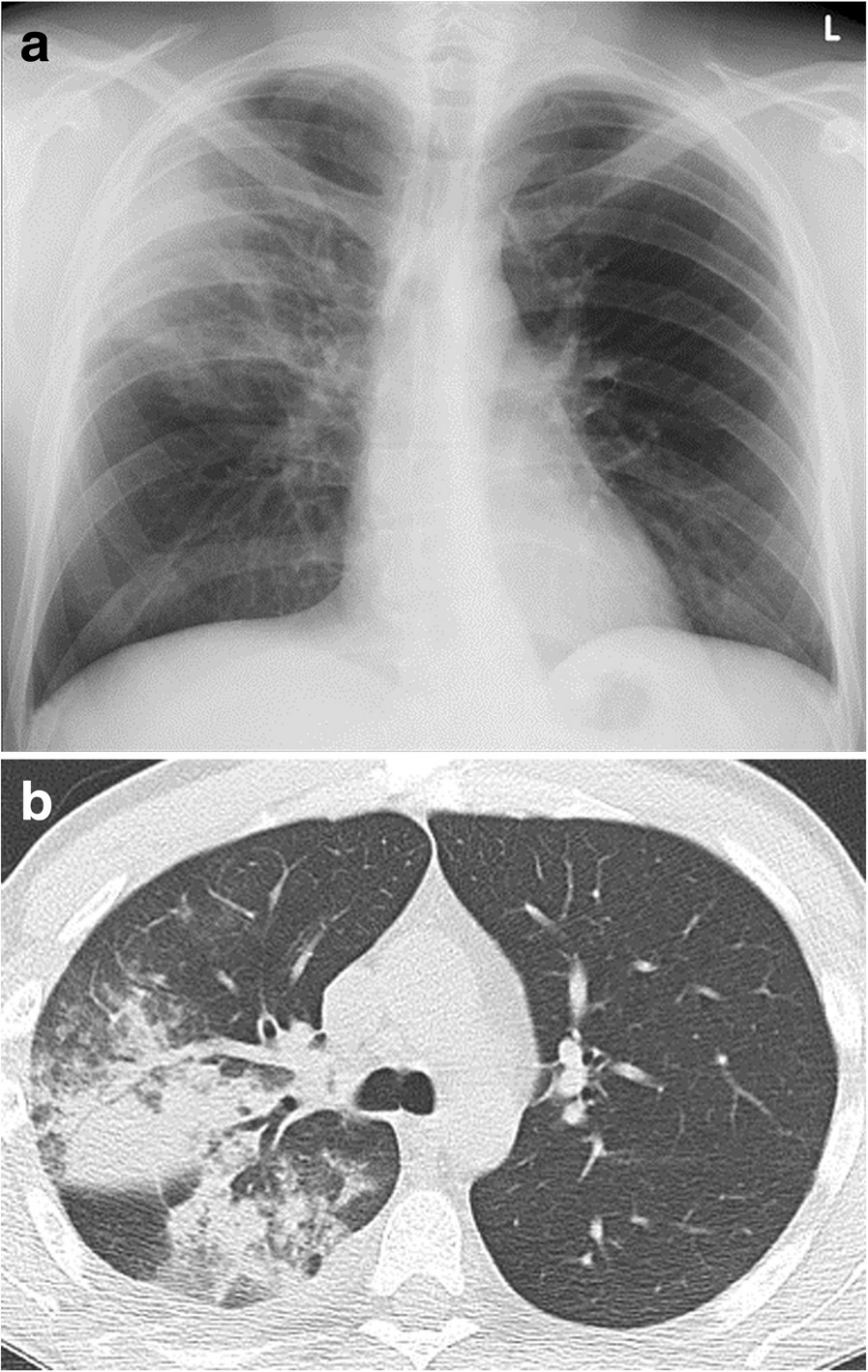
Bacterial pneumonia. Patient with chemotherapy for Burkitt-Lymphoma. a Chest radiograph p.a.: Consolidation projected over the right lateral upper lung zone. b Chest CT at lung window: heterogenous consolidation in the posterior segment of the right upper lobe and the apical segment of the right lower lobe
